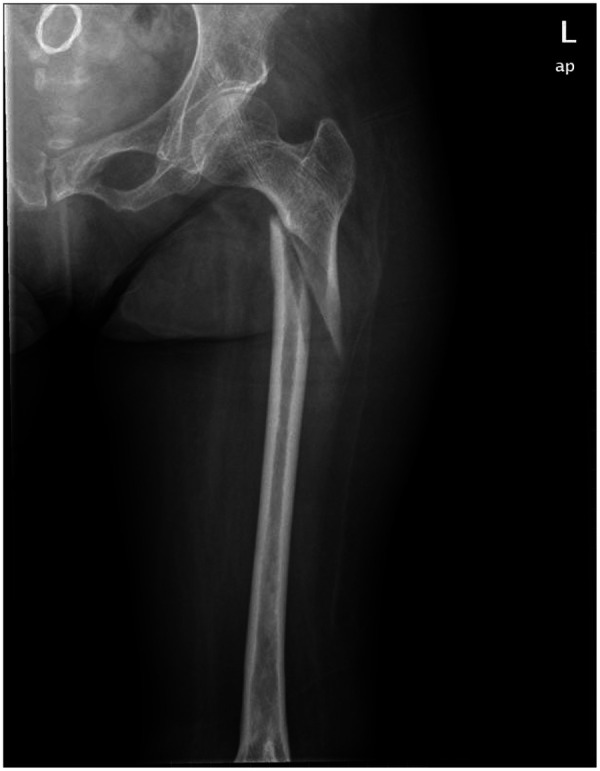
Preoperative anteroposterior x-ray of the left hip showed a left subtrochanteric femoral fracture AO/OTA type A3.
